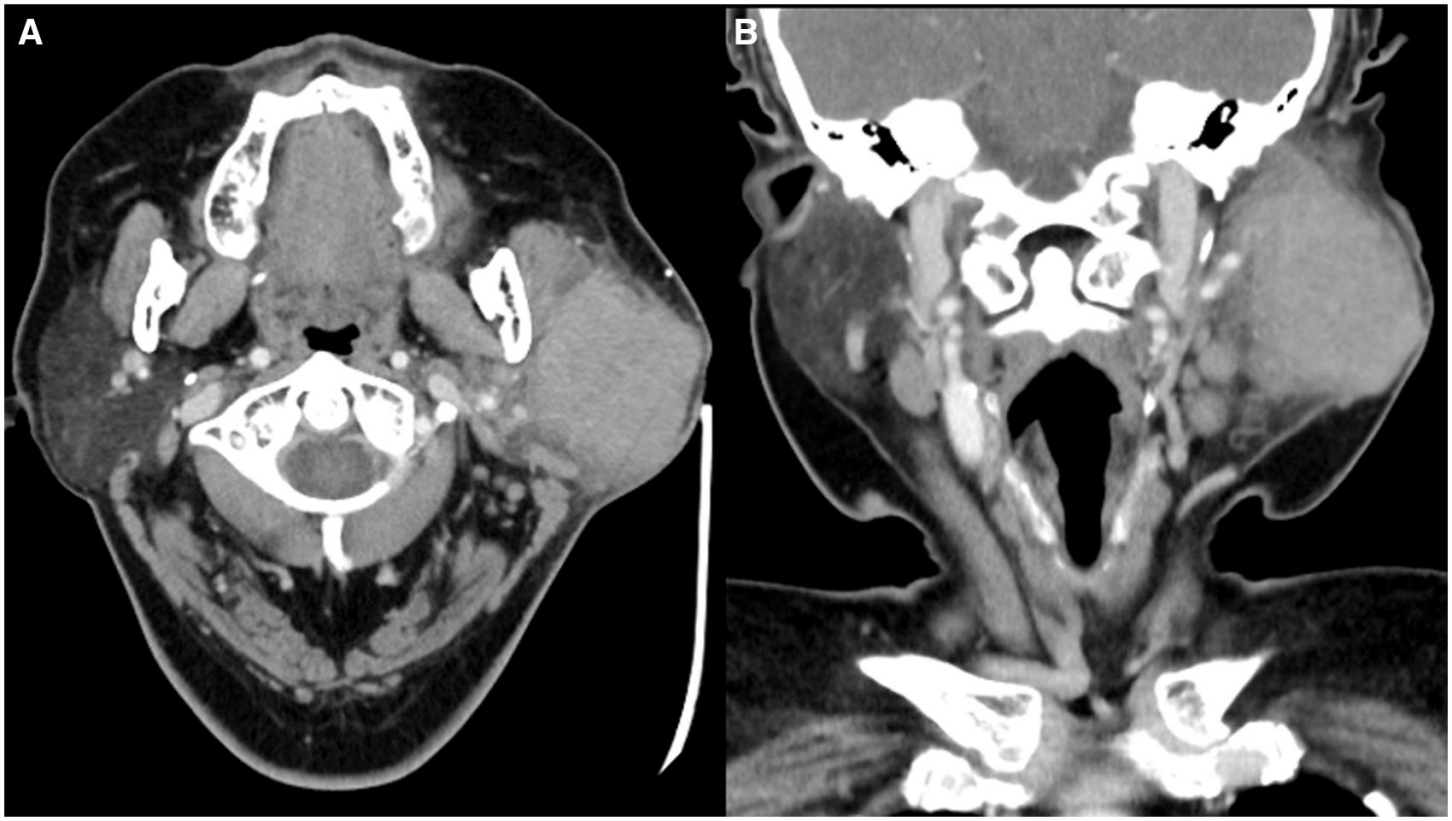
Contrast-enhanced CT ((A) axial; (B) coronal) shows an ill-defined enhancing lesion occupying almost the entire superficial left parotid gland with protrusion into the deep lobe.
